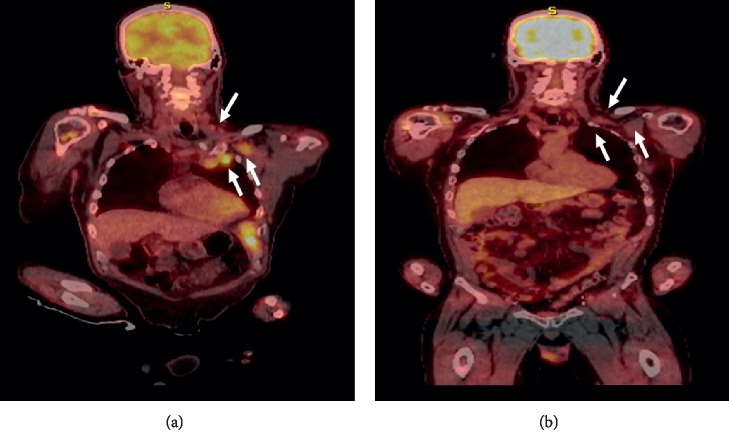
(a) 18F FDG PET-CT at relapse showing left cervical and supraclavicular adenopathy and numerous left subpectoral, axillary, and internal mammary adenopathy (white arrows). (b) 18F FDG PET-CT after 2 cycles of KRD-PACE revealing complete resolution of adenopathy.
